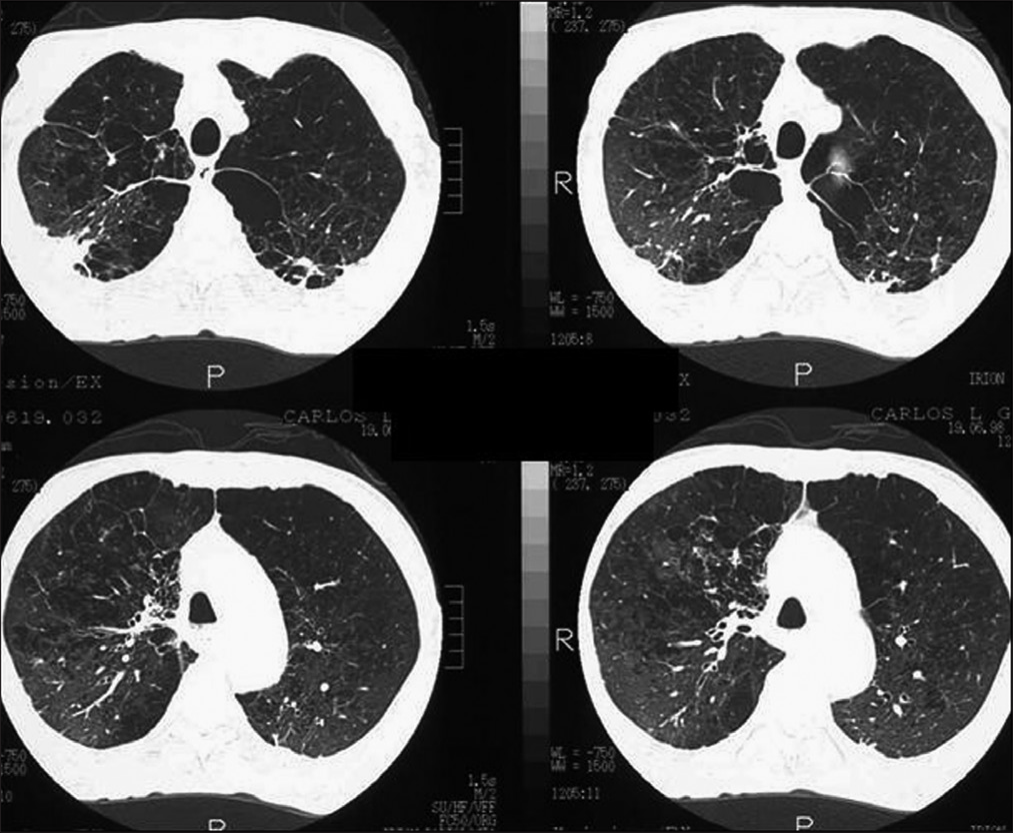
High-resolution computed tomography of a 46-year-old man with acquired immunodeficiency syndrome-related emphysema. This patient was also a smoker and experimented with cocaine and marijuana. A biopsy revealed destruction of the lung parenchyma distal to the terminal bronchioles accompanied by various degrees of inflammation
